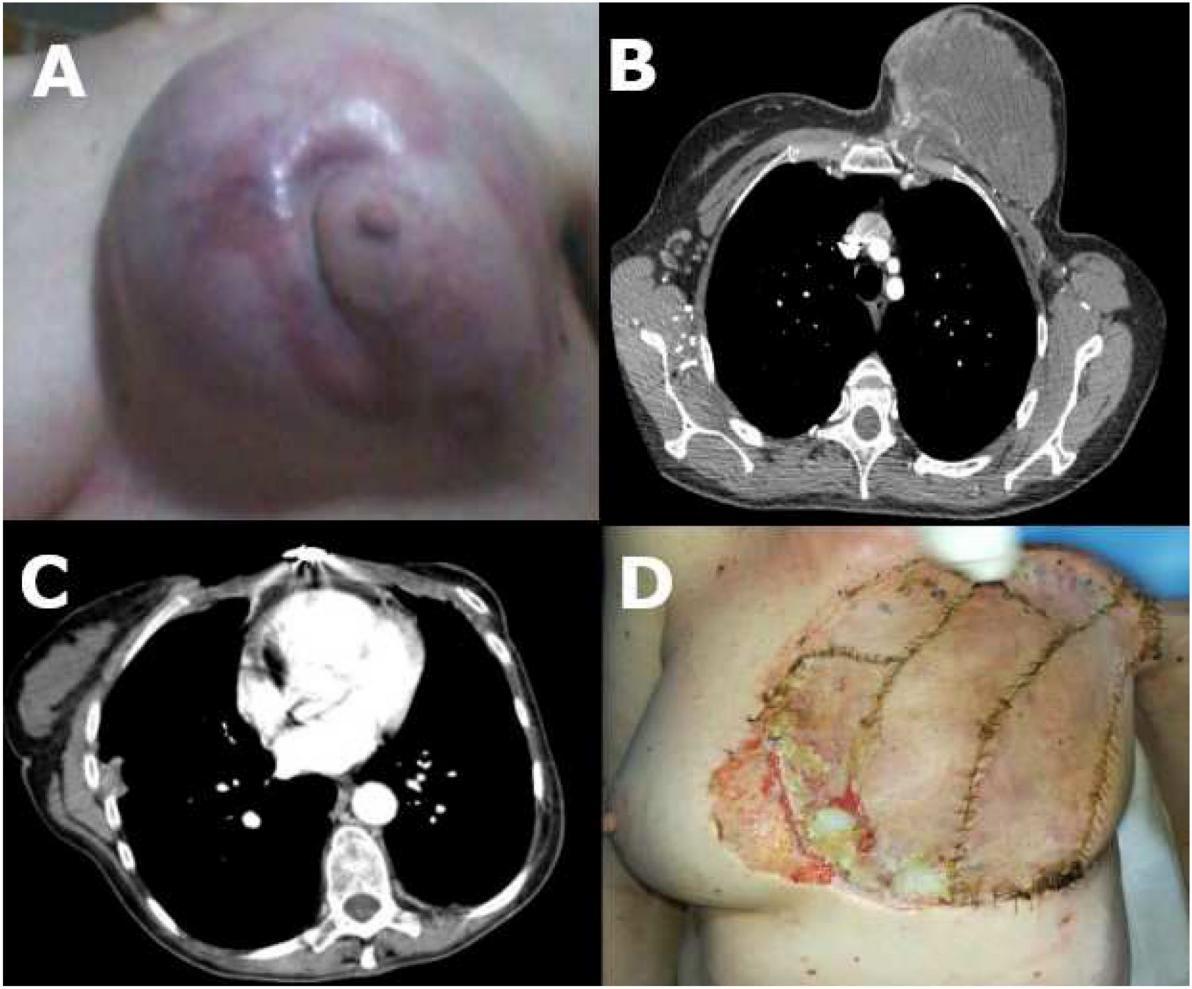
(A) Chest wall sarcoma before surgery; (B) CT scan before surgery; (C) CT scan after surgery; (D) Chest wall sarcoma after surgery with small ulceration (low-right quadrant).
